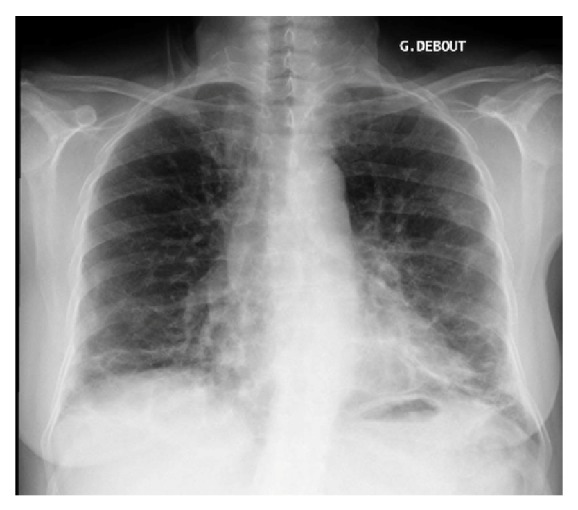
The chest X-ray (in standing position) showing the clear regression of alveolar condensation with some persistent sequelae at both pulmonary bases 1 month after ARDS.
